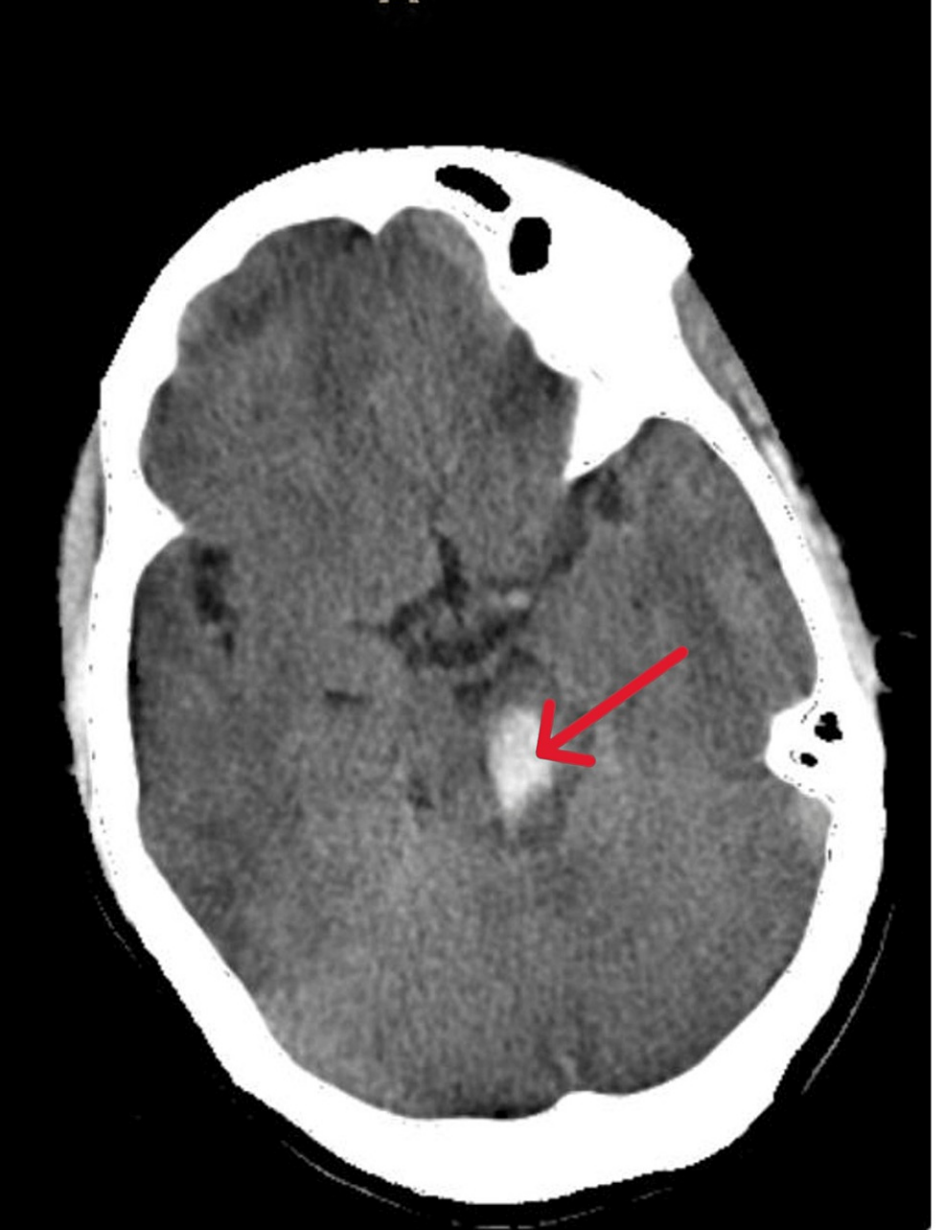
Non-contrast CT brain showing bleed in the mid-brain region Arrow points to the bleed.
